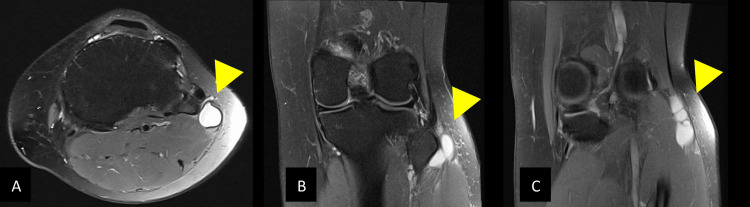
MRI of the left knee demonstrating a multiloculated cystic lesion consistent with a ganglion cyst arising from the proximal tibiofibular joint. (A) Axial T2-weighted fat-suppressed image at the level of the fibular head shows a well-defined, thin-walled, hyperintense ganglion cyst. (B, C) Coronal T2-weighted fat-suppressed images depict the multiloculate cyst extending distally along the head and proximal fibular diaphysis and proximally toward the distal biceps femoris tendon. MRI: magnetic resonance imaging
